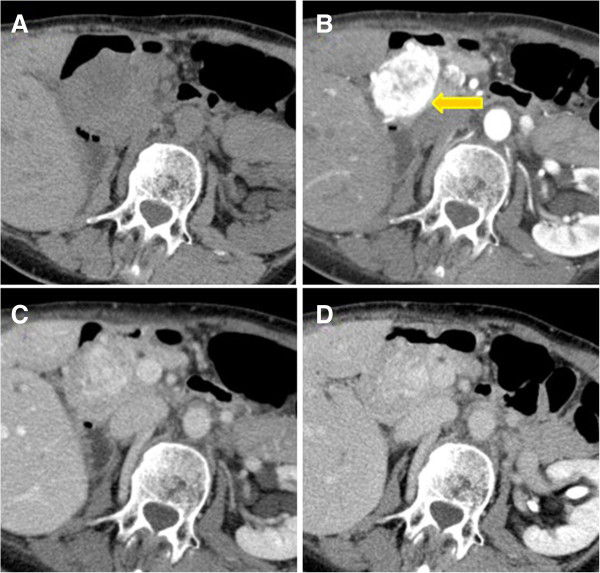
Vascularity pattern. Axial CT scans obtained unenhanced (A) and during arterial (B), portal (C) and delayed (D) phases of contrast enhancement show a large, solitary round metastasis of the pancreatic head. Note that lesion is best seen in the arterial phase and less well seen in portal and delayed phases.
